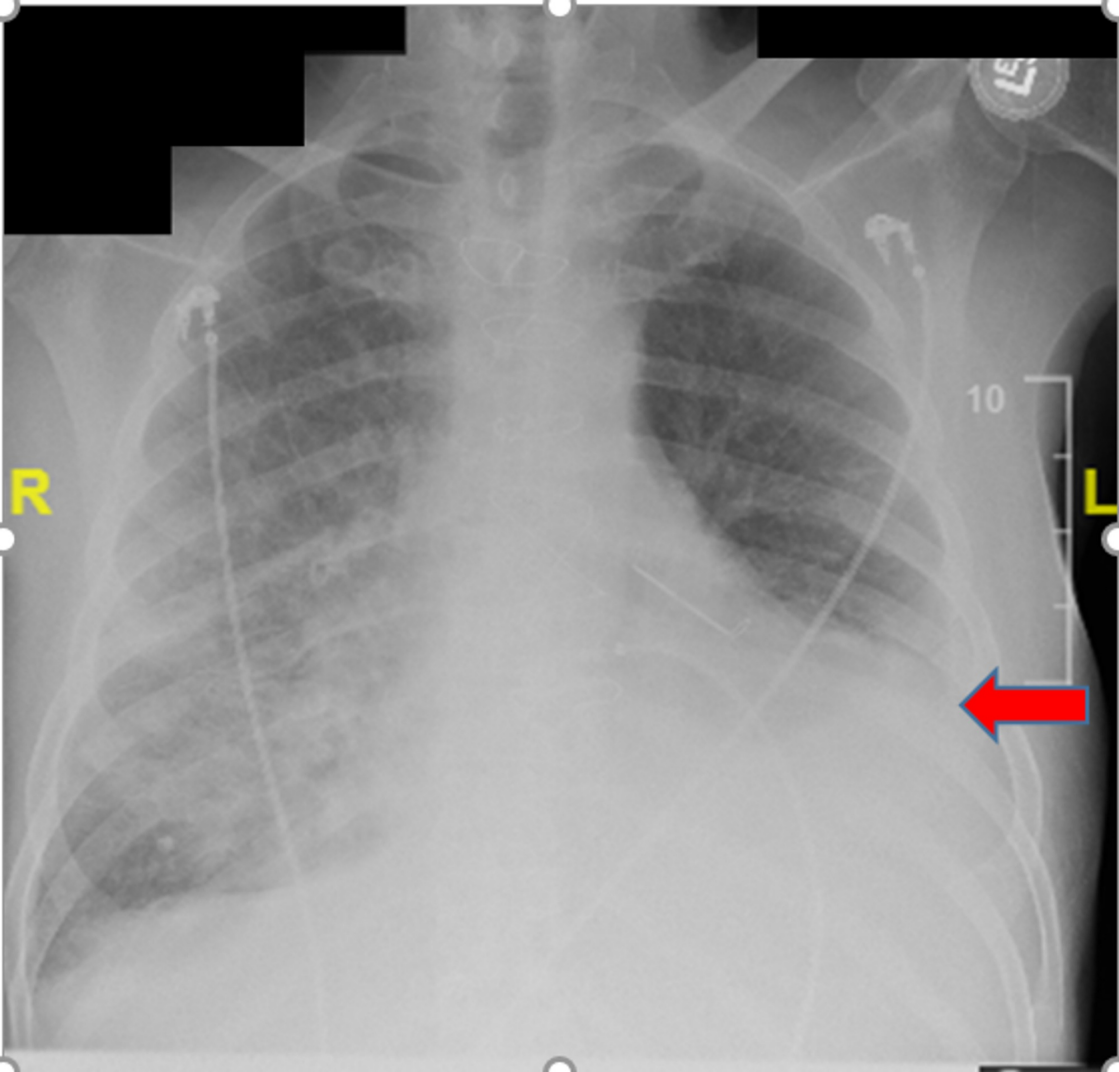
Initial chest X-ray with red arrow pointing at the left sided pleural effusion
